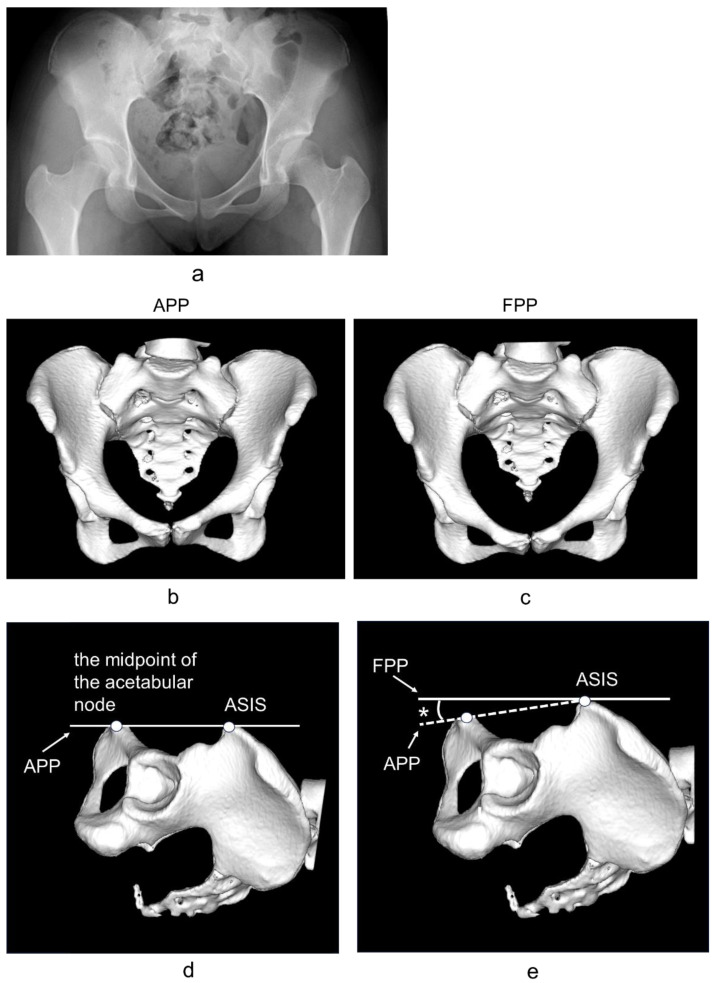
(a) Anteroposterior plain radiograph of the hip joint. (b) Frontal view of a pelvic three-dimensional computed tomography reconstruction obtained by aligning the pelvis with the anterior pelvic plane (APP), which is based on the plane consisting of the left and right anterior superior iliac spines (ASISs) and the midpoint of the acetabular node. (c) Frontal view of a pelvic three-dimensional computed tomography reconstruction obtained by aligning the pelvis with the functional pelvic plane (FPP) (the table plane in the supine position was used as a reference, reflecting the APP sagittal inclination in the supine position). (d) Lateral view of a pelvic three-dimensional computed tomography reconstruction obtained by aligning the pelvis with the anterior pelvic plane (APP), which is based on the plane consisting of the left and right anterior superior iliac spines (ASISs) and the midpoint of the acetabular node. (e) Lateral view of a pelvic three-dimensional computed tomography reconstruction obtained by aligning the pelvis with the functional pelvic plane (FPP) (the table plane in the supine position was used as a reference, reflecting the APP sagittal inclination in the supine position). * Sagittal inclination of the APP in the supine position.
